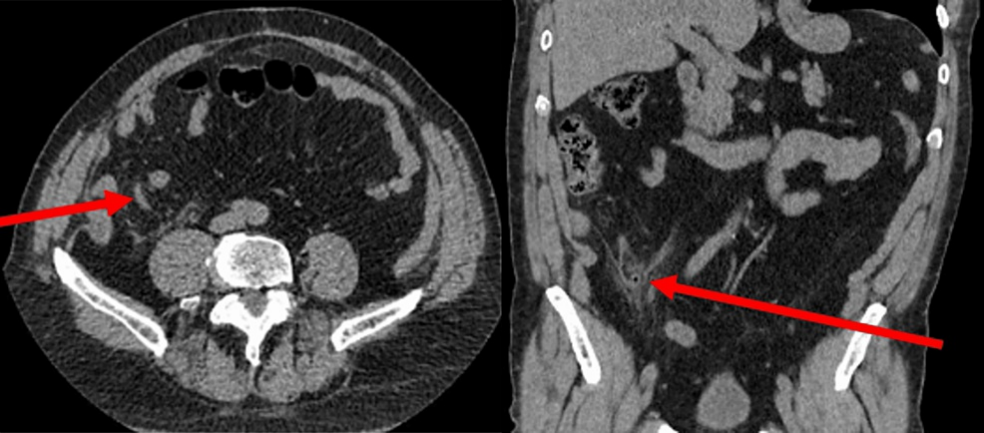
Preoperative computed tomography showing acute uncomplicated appendicitis.
